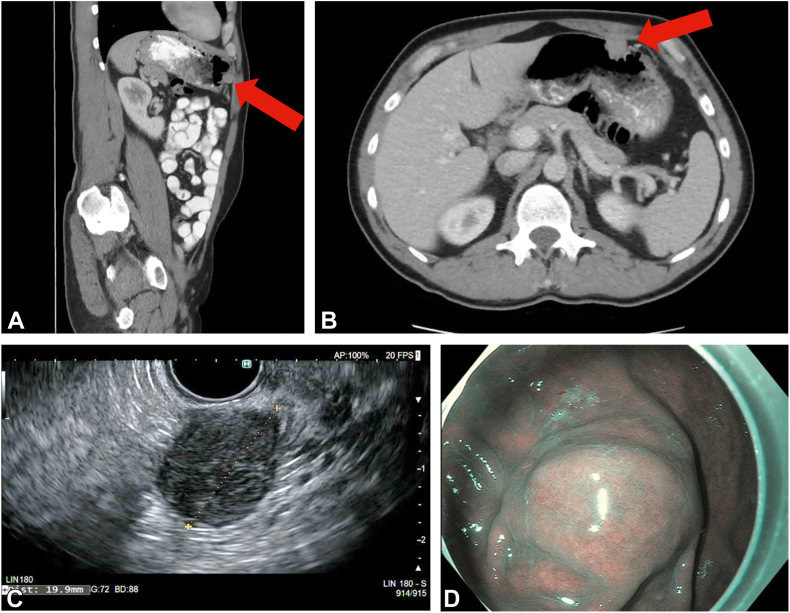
A 2-cm subepithelial lesion located in the anterior wall of the gastric body. A, Sagittal view of an abdominal CT scan showing the lesion (*arrow*). B, Axial view of the abdominal CT scan showing the lesion (*arrow*). C, EUS image of the lesion. D, Endoscopic view of the lesion.
